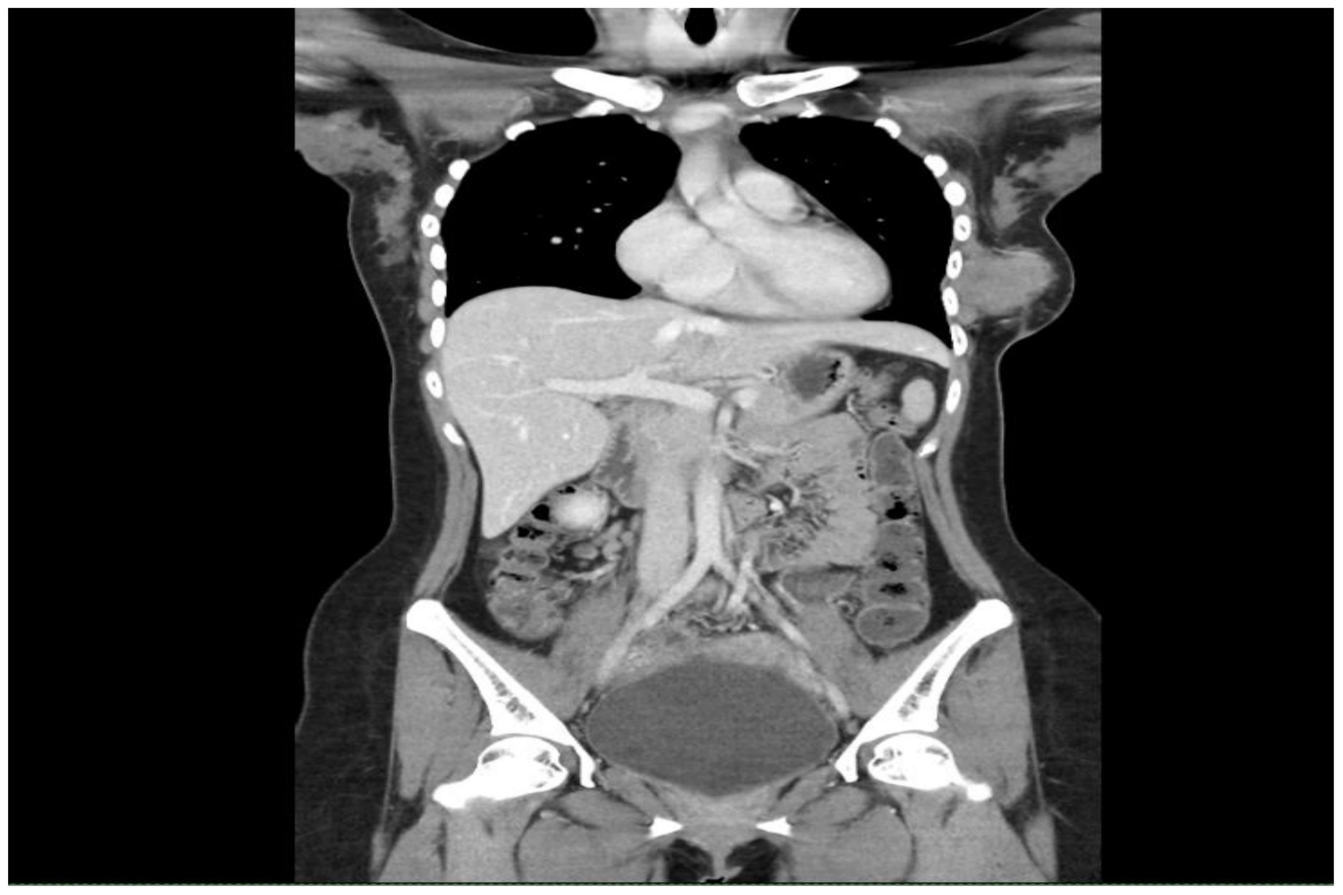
Computed tomography of the thorax, abdomen and pelvis showing the left breast lesion with extension into the pectoralis major, serratus anterior, ribs and pleura.
